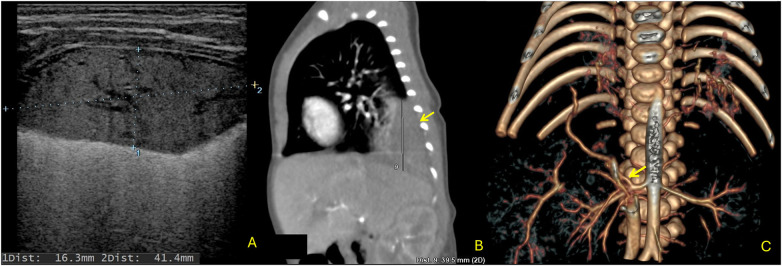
Advanced imaging of the right-sided extralobar pulmonary sequestration. (A) Transthoracic chest ultrasound performed on the first day of life. The image demonstrates a well-defined solid mass measuring 4.1 × 1.6 × 3.9 cm (SD×AP×CC) located in the basal regions of the right lung. (B) Sagittal view of the contrast-enhanced computed tomography (CECT) on the fifth day of life, revealing a large consolidation measuring 3.8 × 2.0 × 5.3 cm (SD×AP×CC) in the lower lobe of the right lung (indicated by the yellow arrow), accompanied by adjacent atelectatic changes. (C) Three-dimensional (3D) CT angiography reconstruction of the vascular supply. The reconstruction clearly delineates the tortuous aberrant systemic feeding artery arising directly from the right renal artery (indicated by the yellow arrow), confirming the diagnosis.
